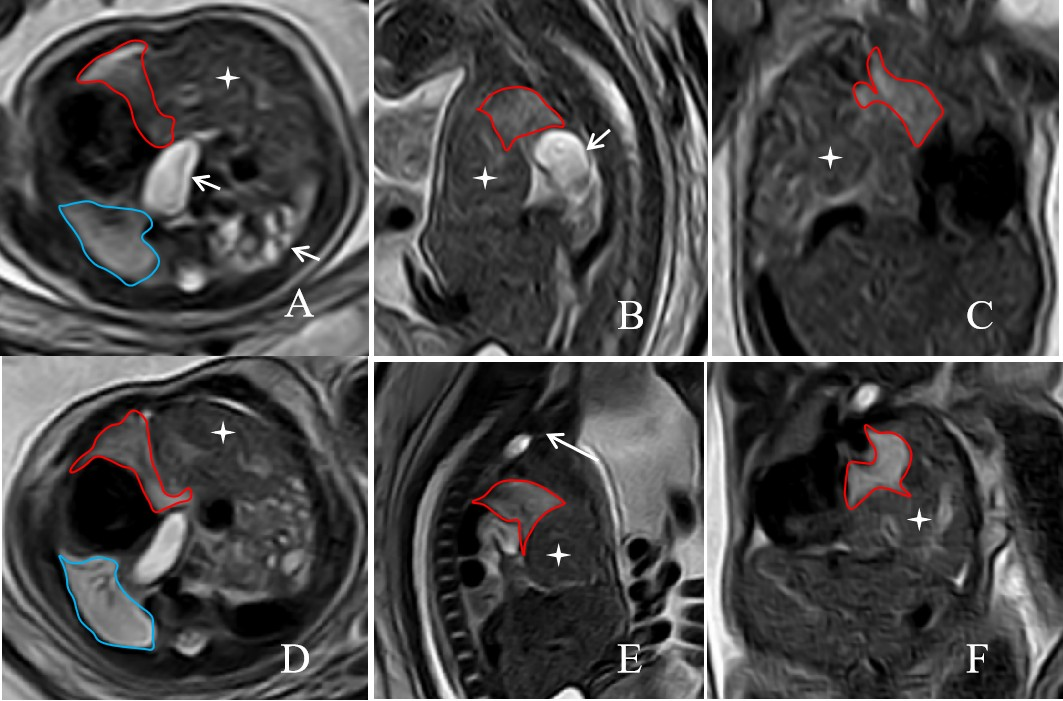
A 33-years-old pregnant woman, the fetus with left CHD on prenatal MRI. The axial, sagittal, and coronal T2-weighted images at 29w3d of gestation(A, B and C) before FETO treatment, and at 34w3d of gestation after FETO treatment(D, E and F) showed abdominal organs, such as the stomach, intestines, and partial left lobe of liver, herniated into the chest cavity(short arrow and cross Star). A T2-weighted image on a sagittal plane showed that a low-signal balloon was inserted into the trachea above the carina during FETO surgery (E, long arrow). The area delineated by the red line represents the left lung that has herniated into the chest cavity; the area outlined by the blue line is the normal right lung.
